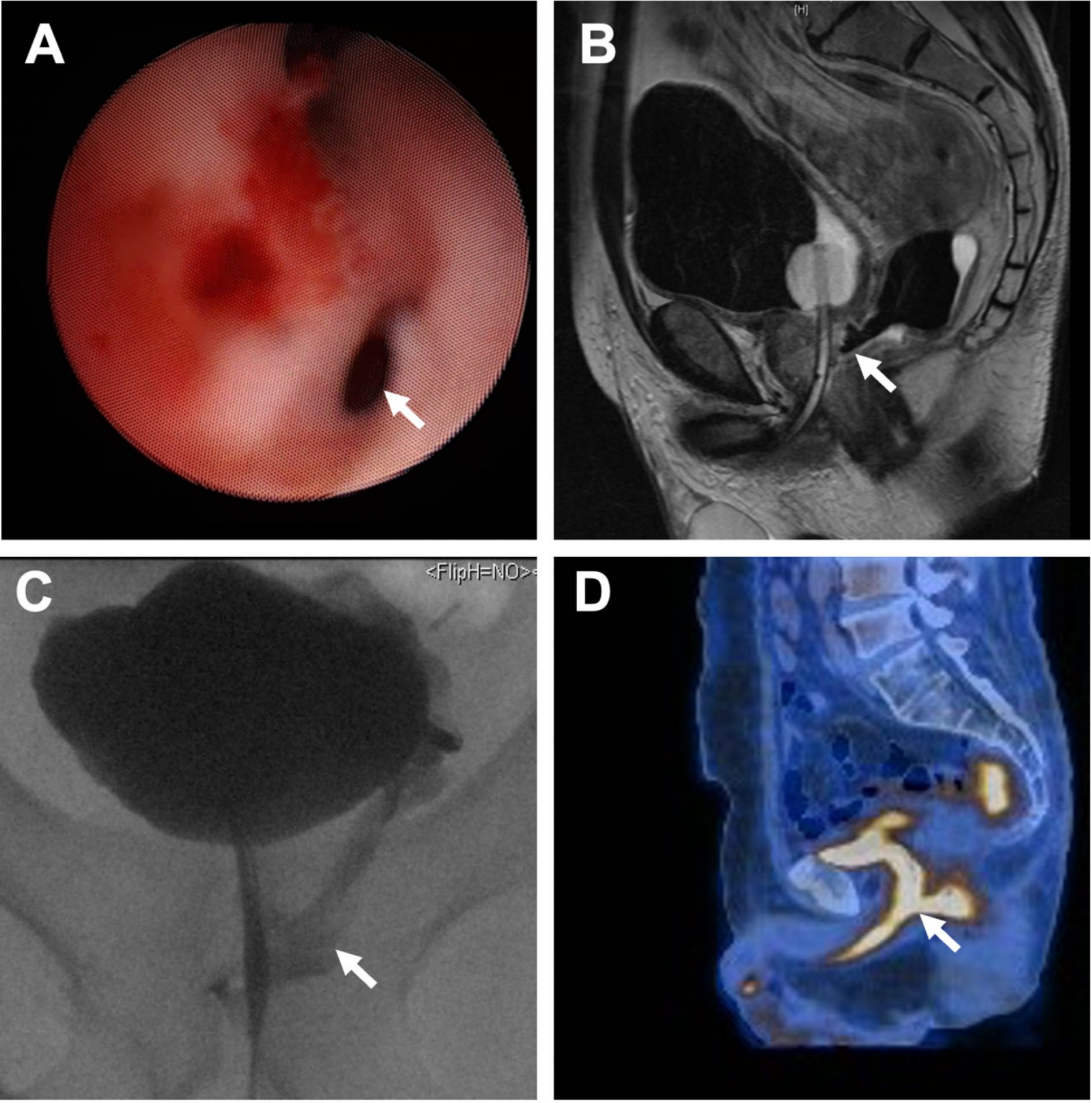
Methods of diagnosis of prostatic fistula. A. Cystoscopic view of the prostatic fistula after TURP (arrow); B. T2 MRI view of recto-prostatic fistula after trans-anal resection for gastrointestinal stromal tumor (arrow); C. Retrograde urethrogram view of the recto-prostatic fistula after colorectal anterior resection and radiotherapy for colorectal cancer; D. PET/CT view of a recto-prostatic fistula with associated urinoma in ischiorectal region following colorectal anterior resection and radiotherapy for colorectal cancer (arrow)
